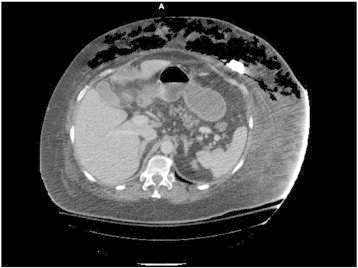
Computed tomography image of extensive subcutaneous emphysema surrounding the IP catheter.
